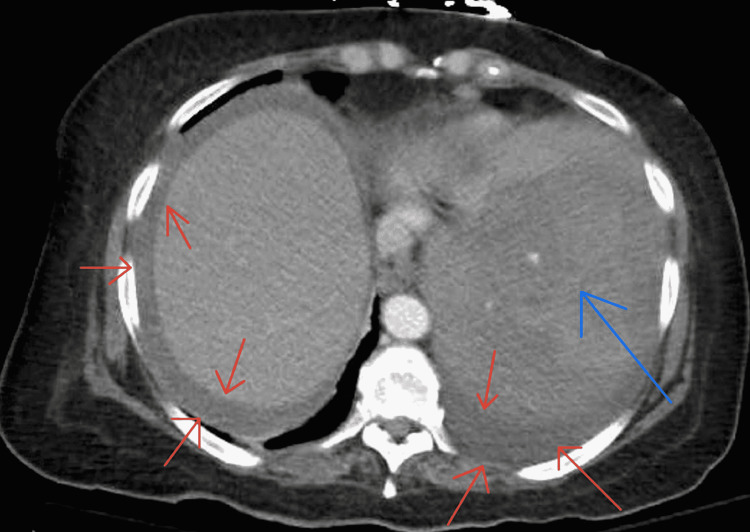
Axial computerized tomography (CT) scan. Red arrows show gross hemoperitoneum. Blue arrow shows splenomegaly.
